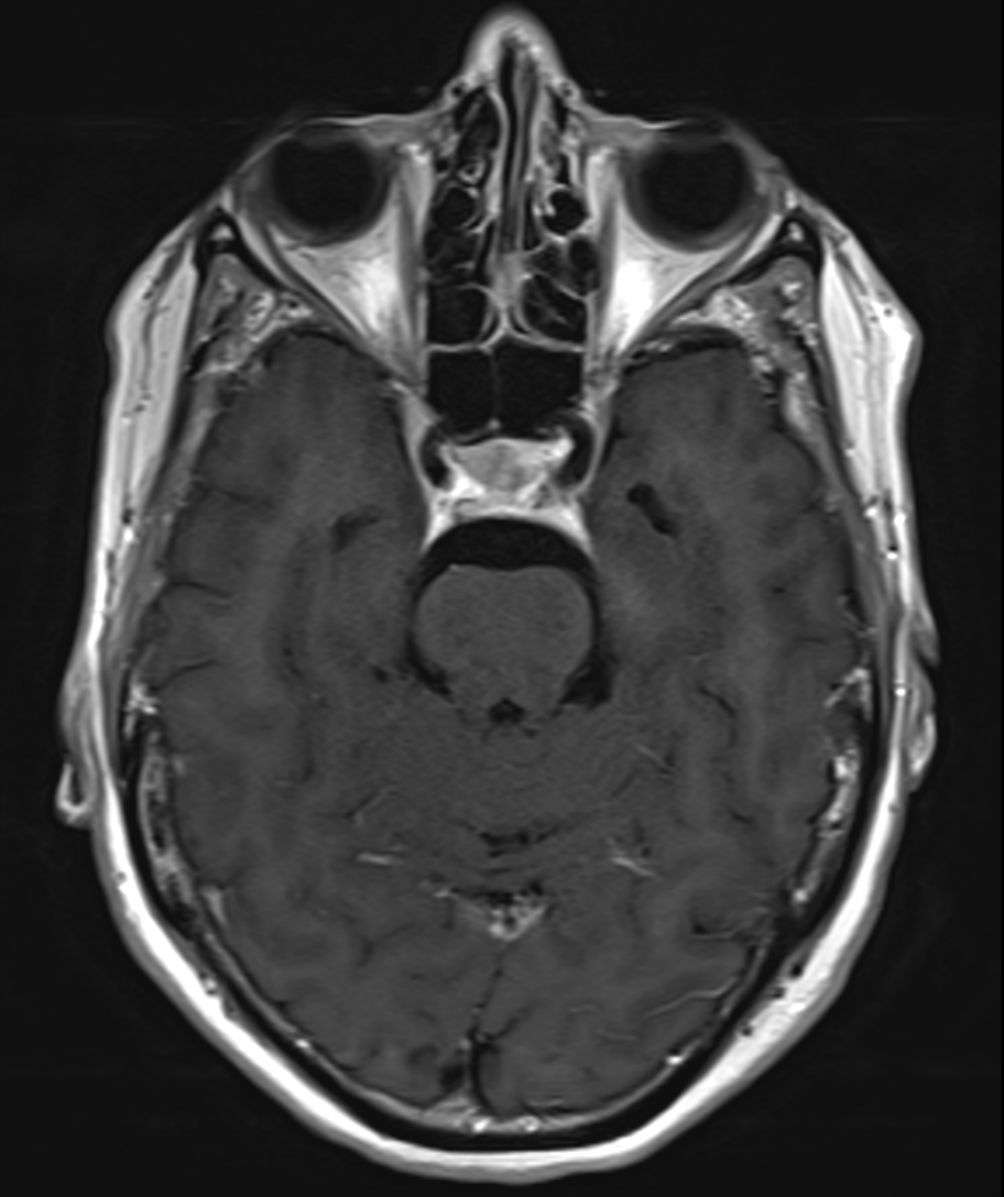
MRI scan (2014) at the level of the cavernous sinus
